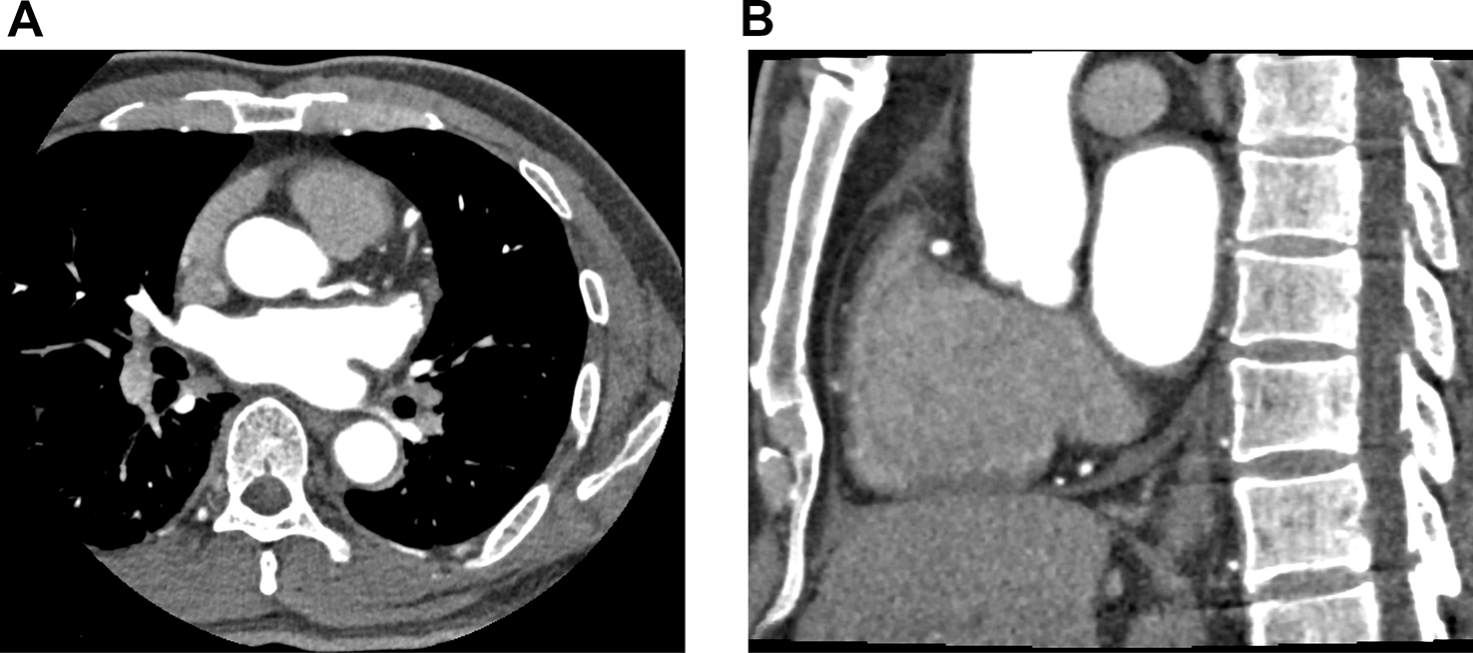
(A) and (B): Repeated coronary CTA before discharge showed no obvious abnormalities
